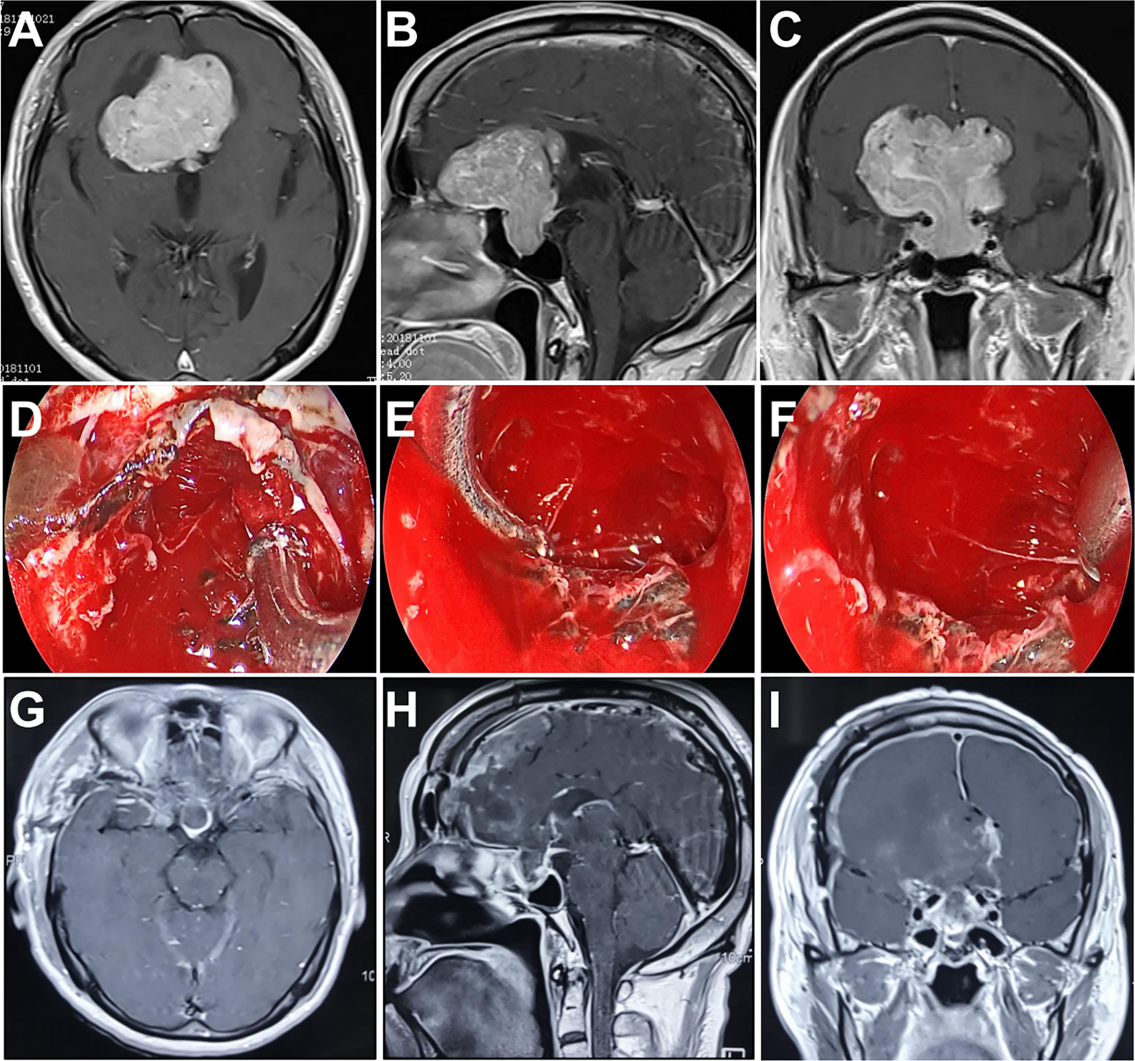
Case 2. Pre- and postoperative imaging findings and intraoperative images. (A–C) Preoperative axial (A), sagittal (B) and coronal (C) gadolinium-enhanced T1-weighted MRI images showing a giant pituitary adenoma with extensive suprasellar extension. (D) Intraoperative photograph showing that the diaphragma sellae is sharply opened and the intrasellar tumor is removed with angled suctions under endoscopic view. (E, F) Intraoperative endoscopic photographs showing the final view of the medial walls of both sides of cavernous sinus as well as the anterior wall of sella turcica after removal of the tumor. (G–I) Postoperative axial (G), sagittal (H) and coronal (I) gadolinium-enhanced T1-weighted MRI images showing gross total resection of the pituitary tumor.
